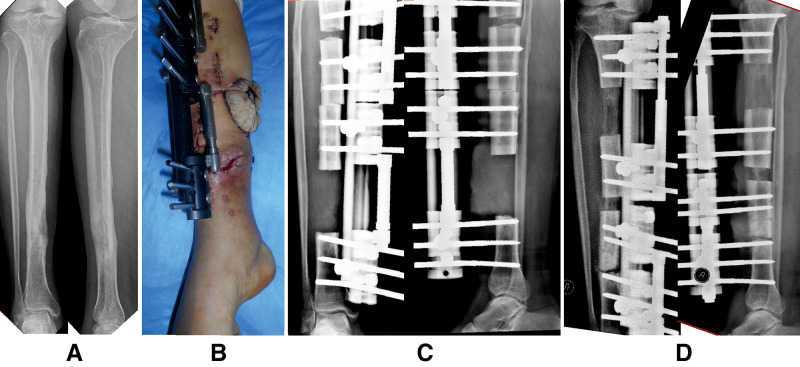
A 53-year-old man suffered chronic osteomyelitis in his right tibia after internal fixation treatment due to a crushing injury caused by a heavy object and was successfully managed by the trifocal bone transport technique (tandem transport, from proximal to distal). (A) Preoperative radiographs indicated that the infectious lesion was located at the distal one-third of the tibial shaft. (B) Removal of devitalized bone and soft tissue by radical debridement; the soft tissue defect was treated by using a local tissue flap. (C) There were 9-cm bone defects, and a trifocal tandem bone transport from proximal to distal was conducted for the limb reconstruction.
